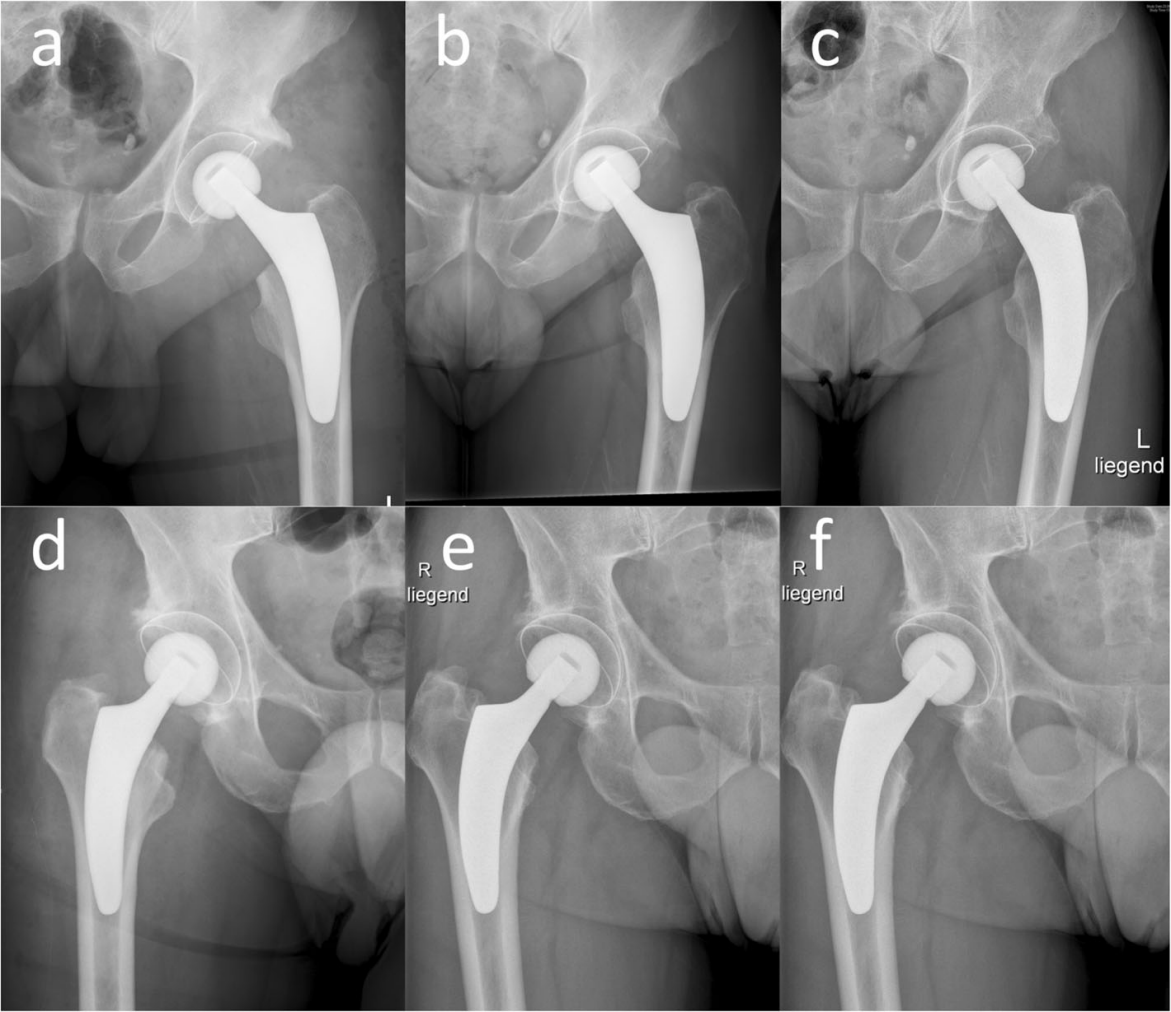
Representative cases without and with 3 mm of subsidence. In this figure, a case, in which no subsidence (a–c) and a case, in which 3 mm of subsidence (d–f) was seen up to 5 years postoperative are presented. Postoperative (a and d), 12 months postoperative (b and e) and 5 years postoperative (c and f) a-p x-rays are depicted
